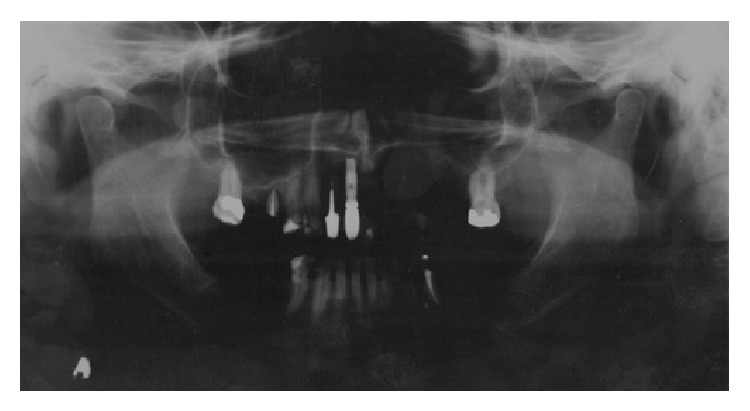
Initial panoramic radiography (August 2008).
